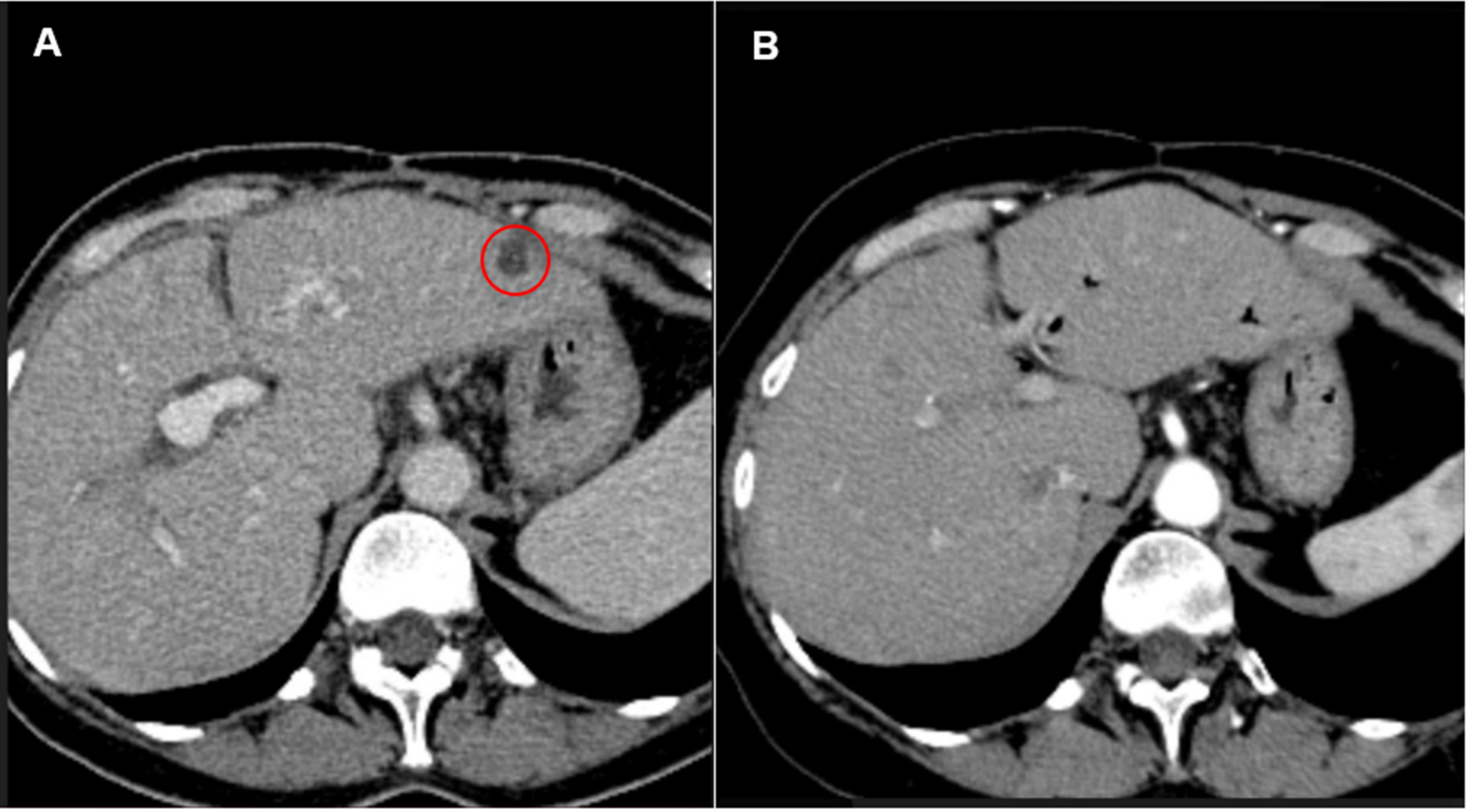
(A) CT scan of S2 liver lesion, 10 mm in greatest dimension before talazoparib treatment (March 2019), (B) CT scan of S2 of the liver after 25 months of treatment without visual evidence of the disease (April 2021).
